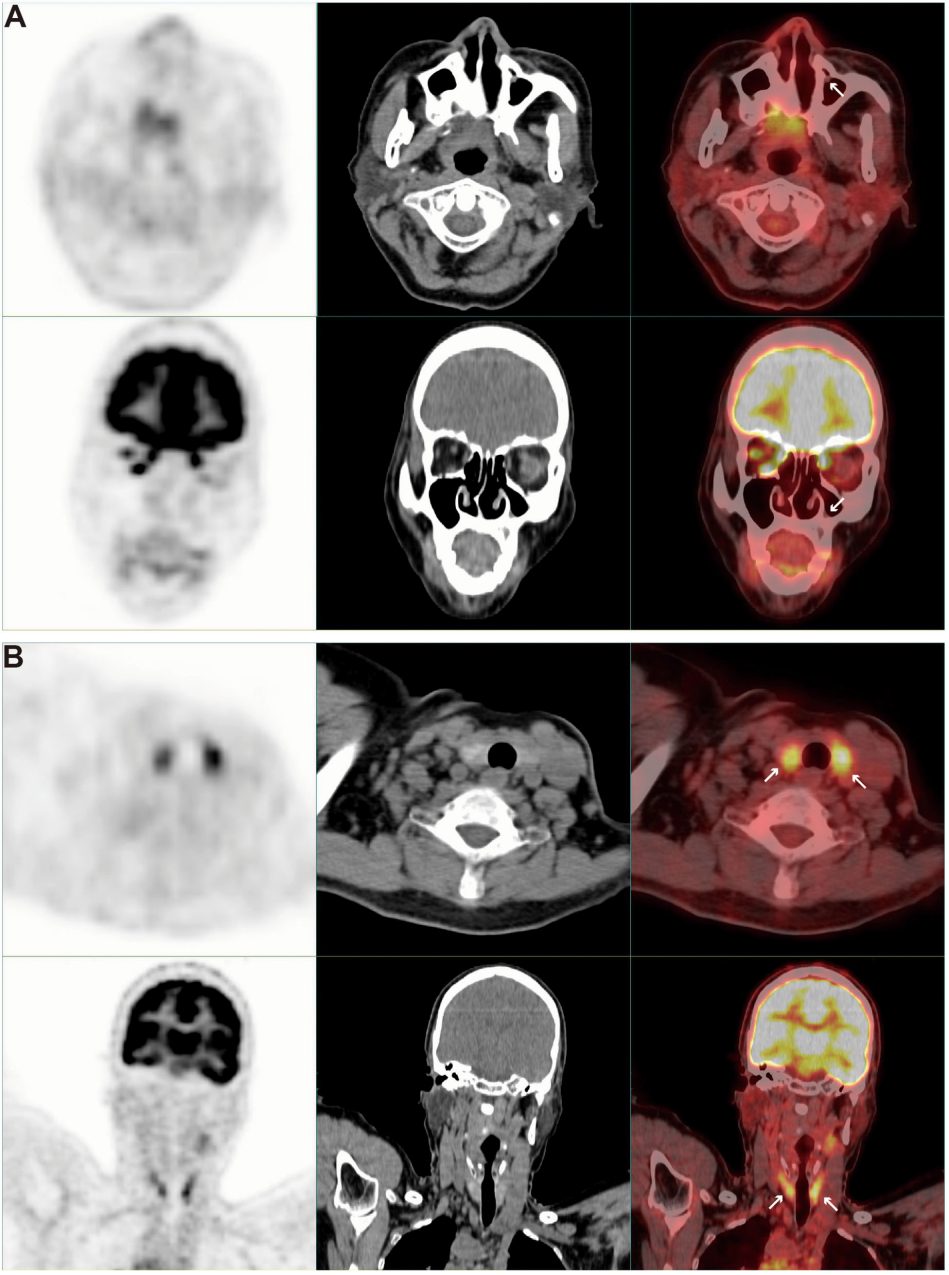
PET / CT scan showed abnormalities of maxillary sinus and thyroid. (A) Axial and coronal PET, CT, and PET/CT fusion images show mucinous changes in the left maxillary sinus. (B) Axial and coronal PET, CT, and PET/CT fusion images showed abnormal tracer uptake in the patient's thyroid.
